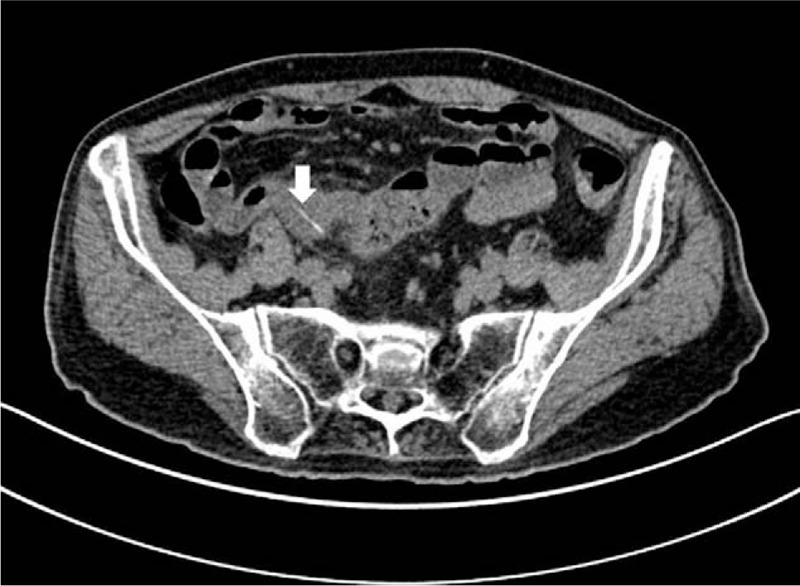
Fish bone perforating small intestinal on CT image. Axial noncontrast CT image demonstrating an intestinal foreign body (arrow) with associated small intestinal wall thickening and edema. The high-density foreign body with a diameter of 2.9 mm was found to represent aluminum pill packaging. Note also the surrounding mesenteric inflammatory fat stranding.
